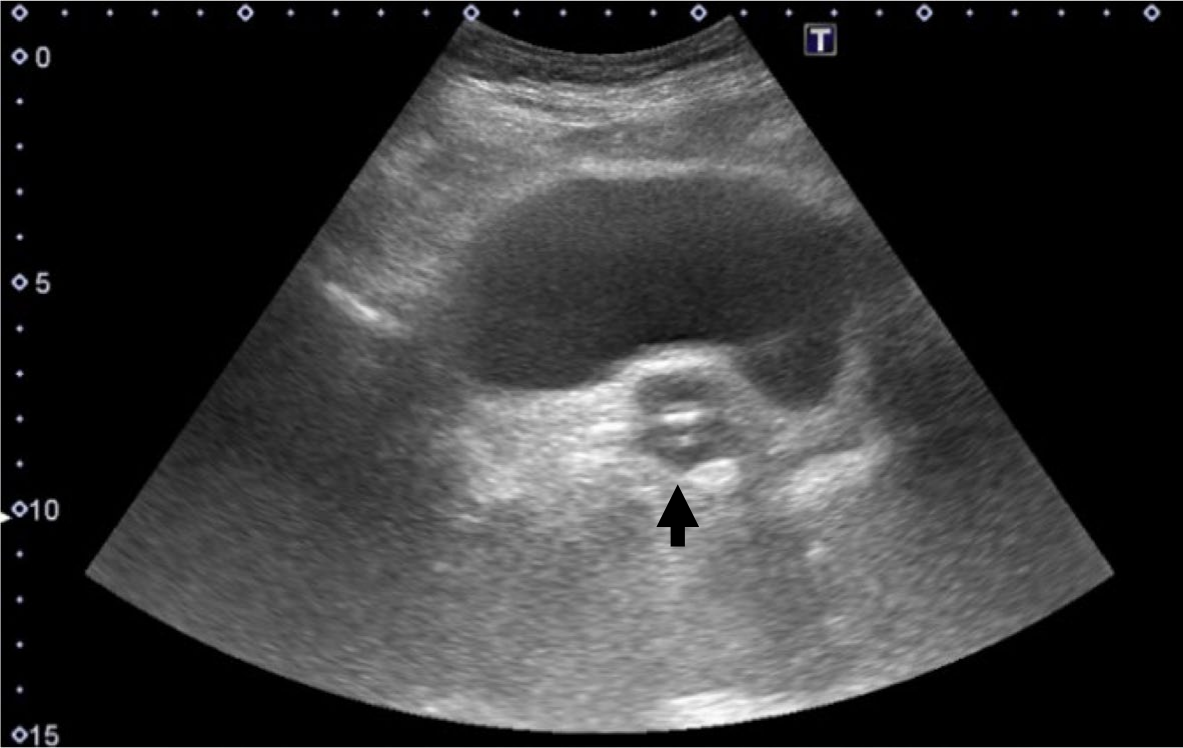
Transverse ultrasound image of the bladder showing a urethral catheter balloon (arrow) on the dorsal side of the bladder
